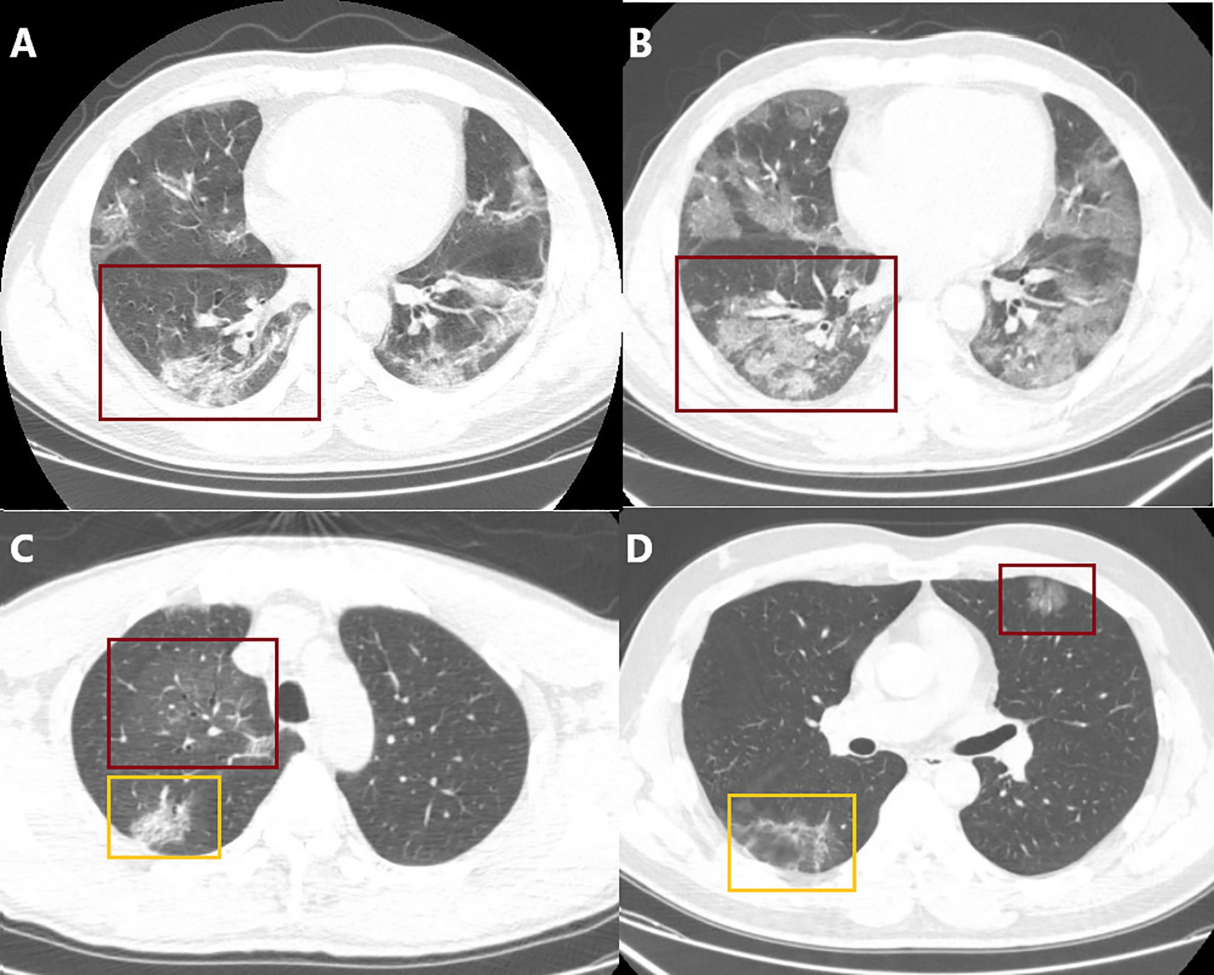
Typical CT manifestations 2. A and B. An initial CT scan (A) compared with a follow-up scan 27 hours later (B) showed that the GGO co-existed with consolidations (red frame) and progressed rapidly. The scope was significantly expanded, and consolidative lesions increased. The initial semiquantitative scores were left lung = 14, and right lung = 13, totaling 27; the follow-up semiquantitative scores were left lung = 19, and right lung = 17, totaling 36. C. The light density of GGO (red frame) with multiple air bronchogram, or more appropriately, bronchiectasis. Subpleural reticular pattern (yellow frame) in the apical segment of the right superior lobe. D. GGO (red frame) and reticular pattern (yellow frame) are located in the subpleural area of the left and right lung, respectively.
